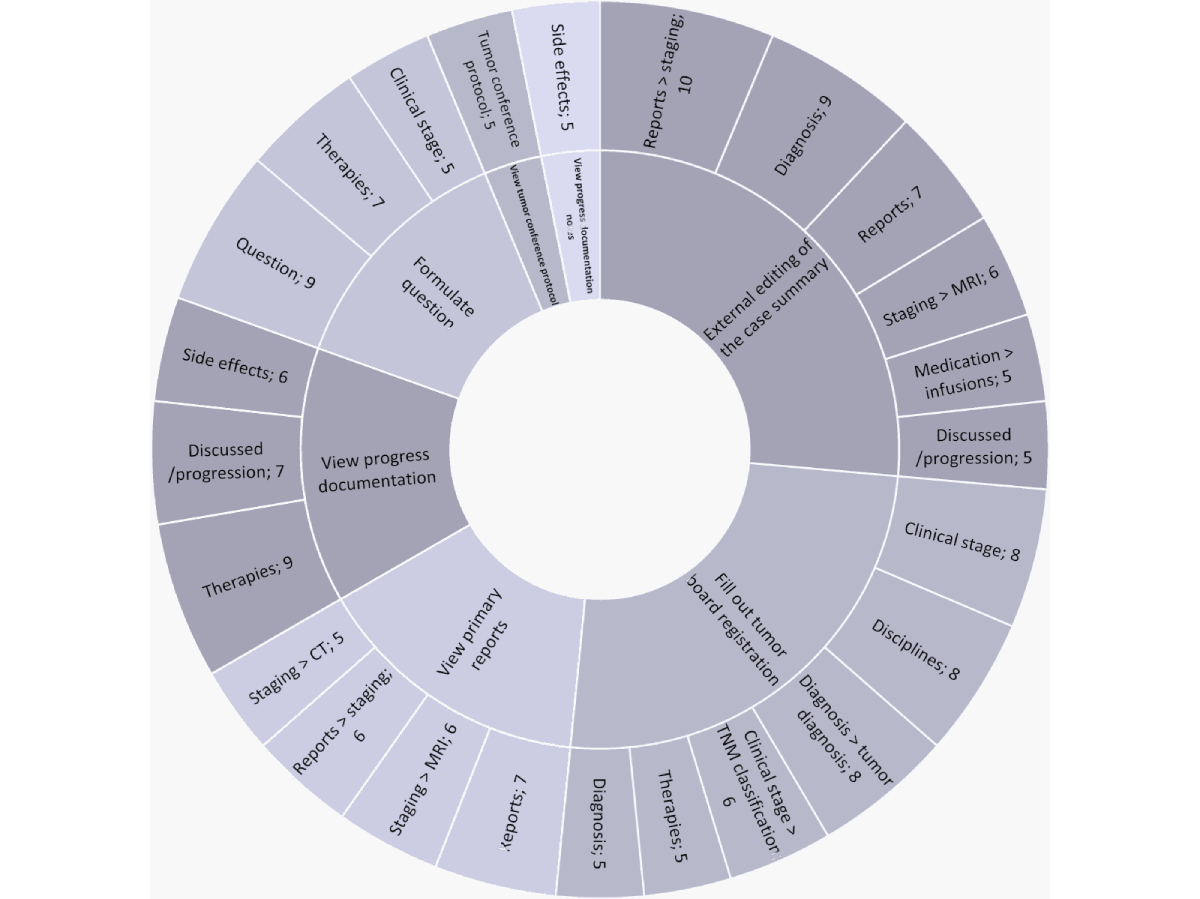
The work steps, which are located within the inner circle, are accompanied by the associated information, which is presented in the outer circle. Additionally, the number of code-overlaps in the transcripts is indicated by the numbers within the outer circle. Only those overlaps occurring in transcripts at least 5 times are included. CT: computed tomography; MRI: magnetic resonance imaging and computed tomography.
